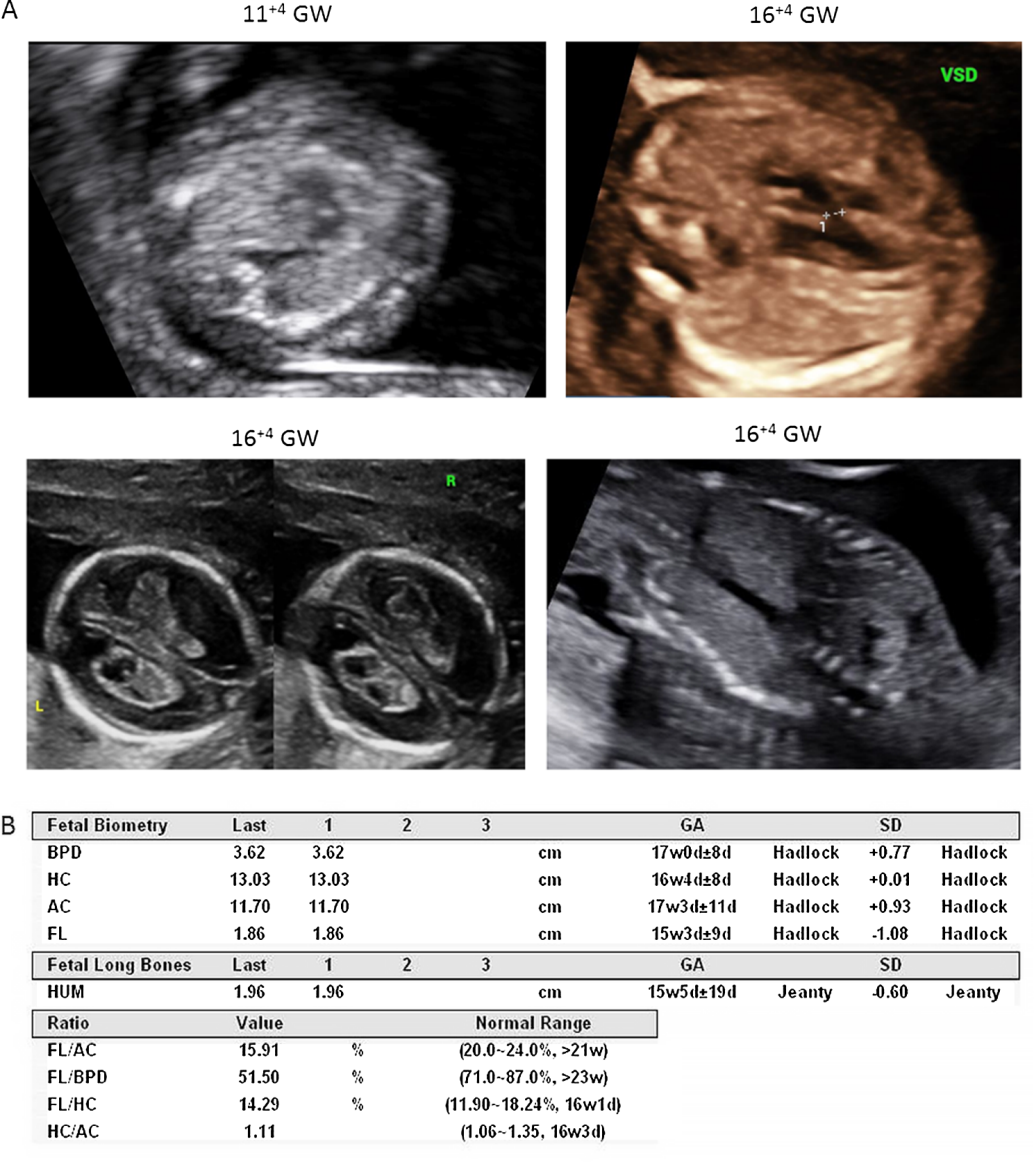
Fetal abnormalities found by sonographic scanning. A Sonographic scanning detected ventricular septal defect at 11 + 4 GW and VSD of 0.02 cm, bilateral choroid plexus cysts of the brain and hyperechogenic kidneys at 16 + 4 GW. B The fetal biometry including BPD, HC, AC, FL and HL, the ratio of FL/AC, FL/BPD, FL/HC, HC/AC were all in the normal range. BPD: biparietal diameter; HC: head circumference; AC: abdominal circumference; FL: femur length; HL: humerus length
